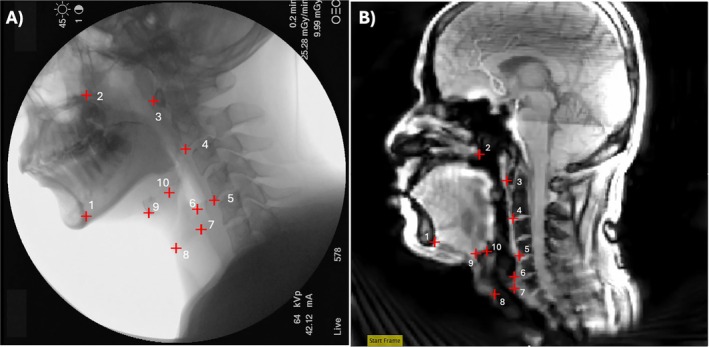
The landmarks used in Computational Analysis of Swallowing Mechanics (CASM). (A) The 10 anatomical landmarks tracked via CASM in VFSS. (B) The 10 anatomical landmarks tracked via CASM in dynamic MRI. Images have been rotated upright for the ease of the readers.
